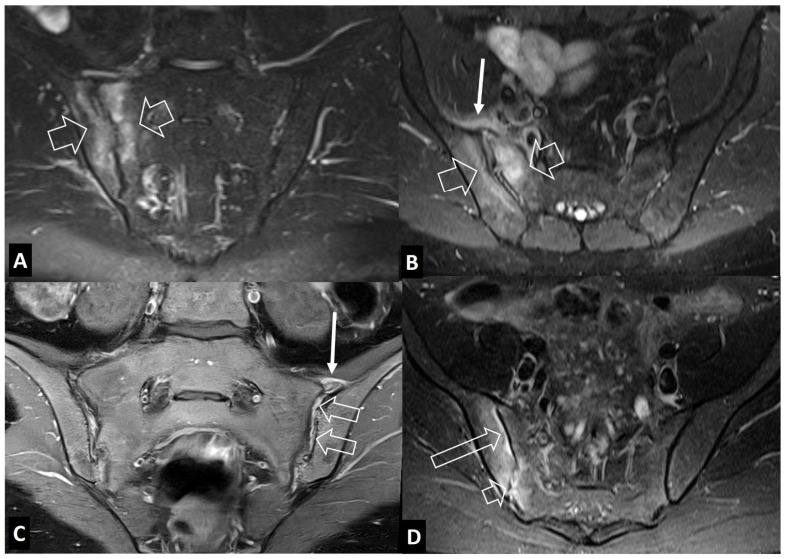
Oblique coronal (A) and oblique axial (B) STIR MR images in a 45-year-old male patient with infectious sacroiliitis showing subarticular bone marrow edema (open arrows), high intra-articular signal intensity and soft-tissue edema anterior to the joint (thin arrow). (C) Oblique coronal fat-suppressed contrast-enhanced T1-w MR image in a 32-year-old female patient with psoriatic axial spondyloarthropathy, showing intra-articular enhancement (open arrows) and anterior capsulitis (arrow). (D) Oblique axial STIR MR image in a 24-year-old female patient with a history of Familial Mediterranean fever and recent respiratory tract infections, showing subarticular bone marrow edema (long open arrow) and high intra-articular signal intensity (short open arrow), in keeping with reactive arthritis.
